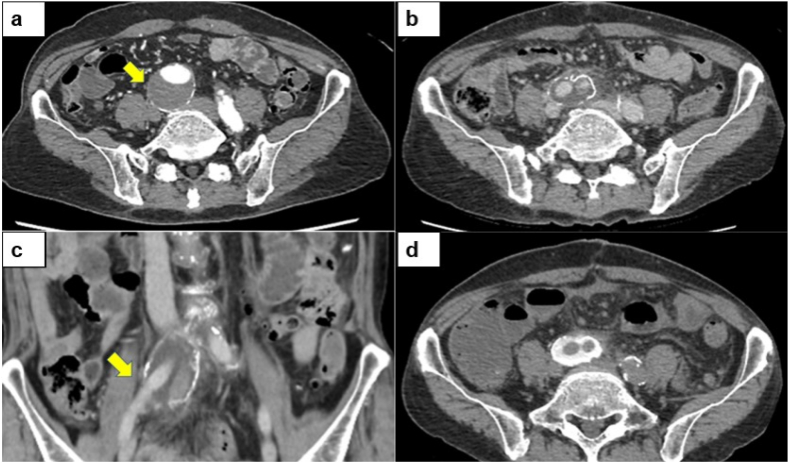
(a) Preoperative contrast-enhanced CT image showing the right common iliac aneurysmal sac adjacent to the right ureter (arrow). (b), (c) Postoperative contrast CT image showing fluid-filled right common iliac aneurysmal sac. The arrow indicates the right ureter. (d) Plain CT revealed accumulation of contrast medium within the right common iliac aneurysmal sac.
